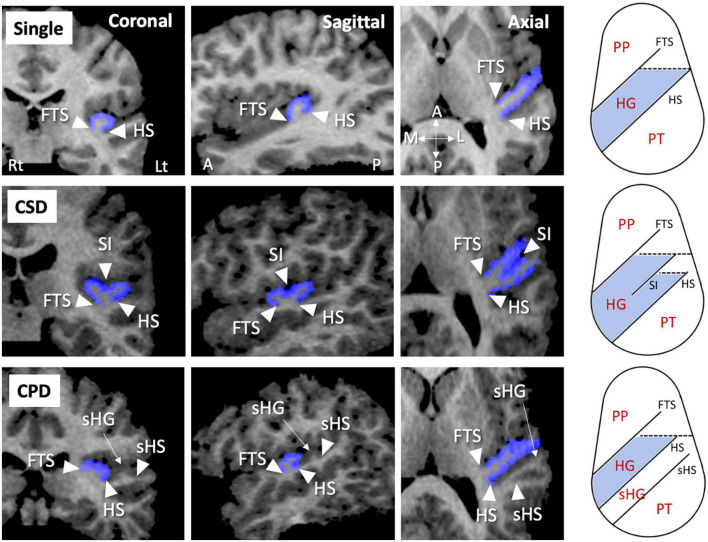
Sample MR images of different gyrification pattern in the Heschl’s gyrus (HG) (colored in blue). These HG patterns have been demonstrated also in our previous publications (22–26). A, anterior; CPD, complete posterior duplication; CSD, common stem duplication; FTS, first transverse sulcus; HS, Heschl’s sulcus; L, lateral; Lt, left; P, posterior; M, medial; PP, planum polare; Rt, right; sHG, second Heschl’s gyrus; sHS, second Heschl’s sulcus; SI, sulcus intermedius.
